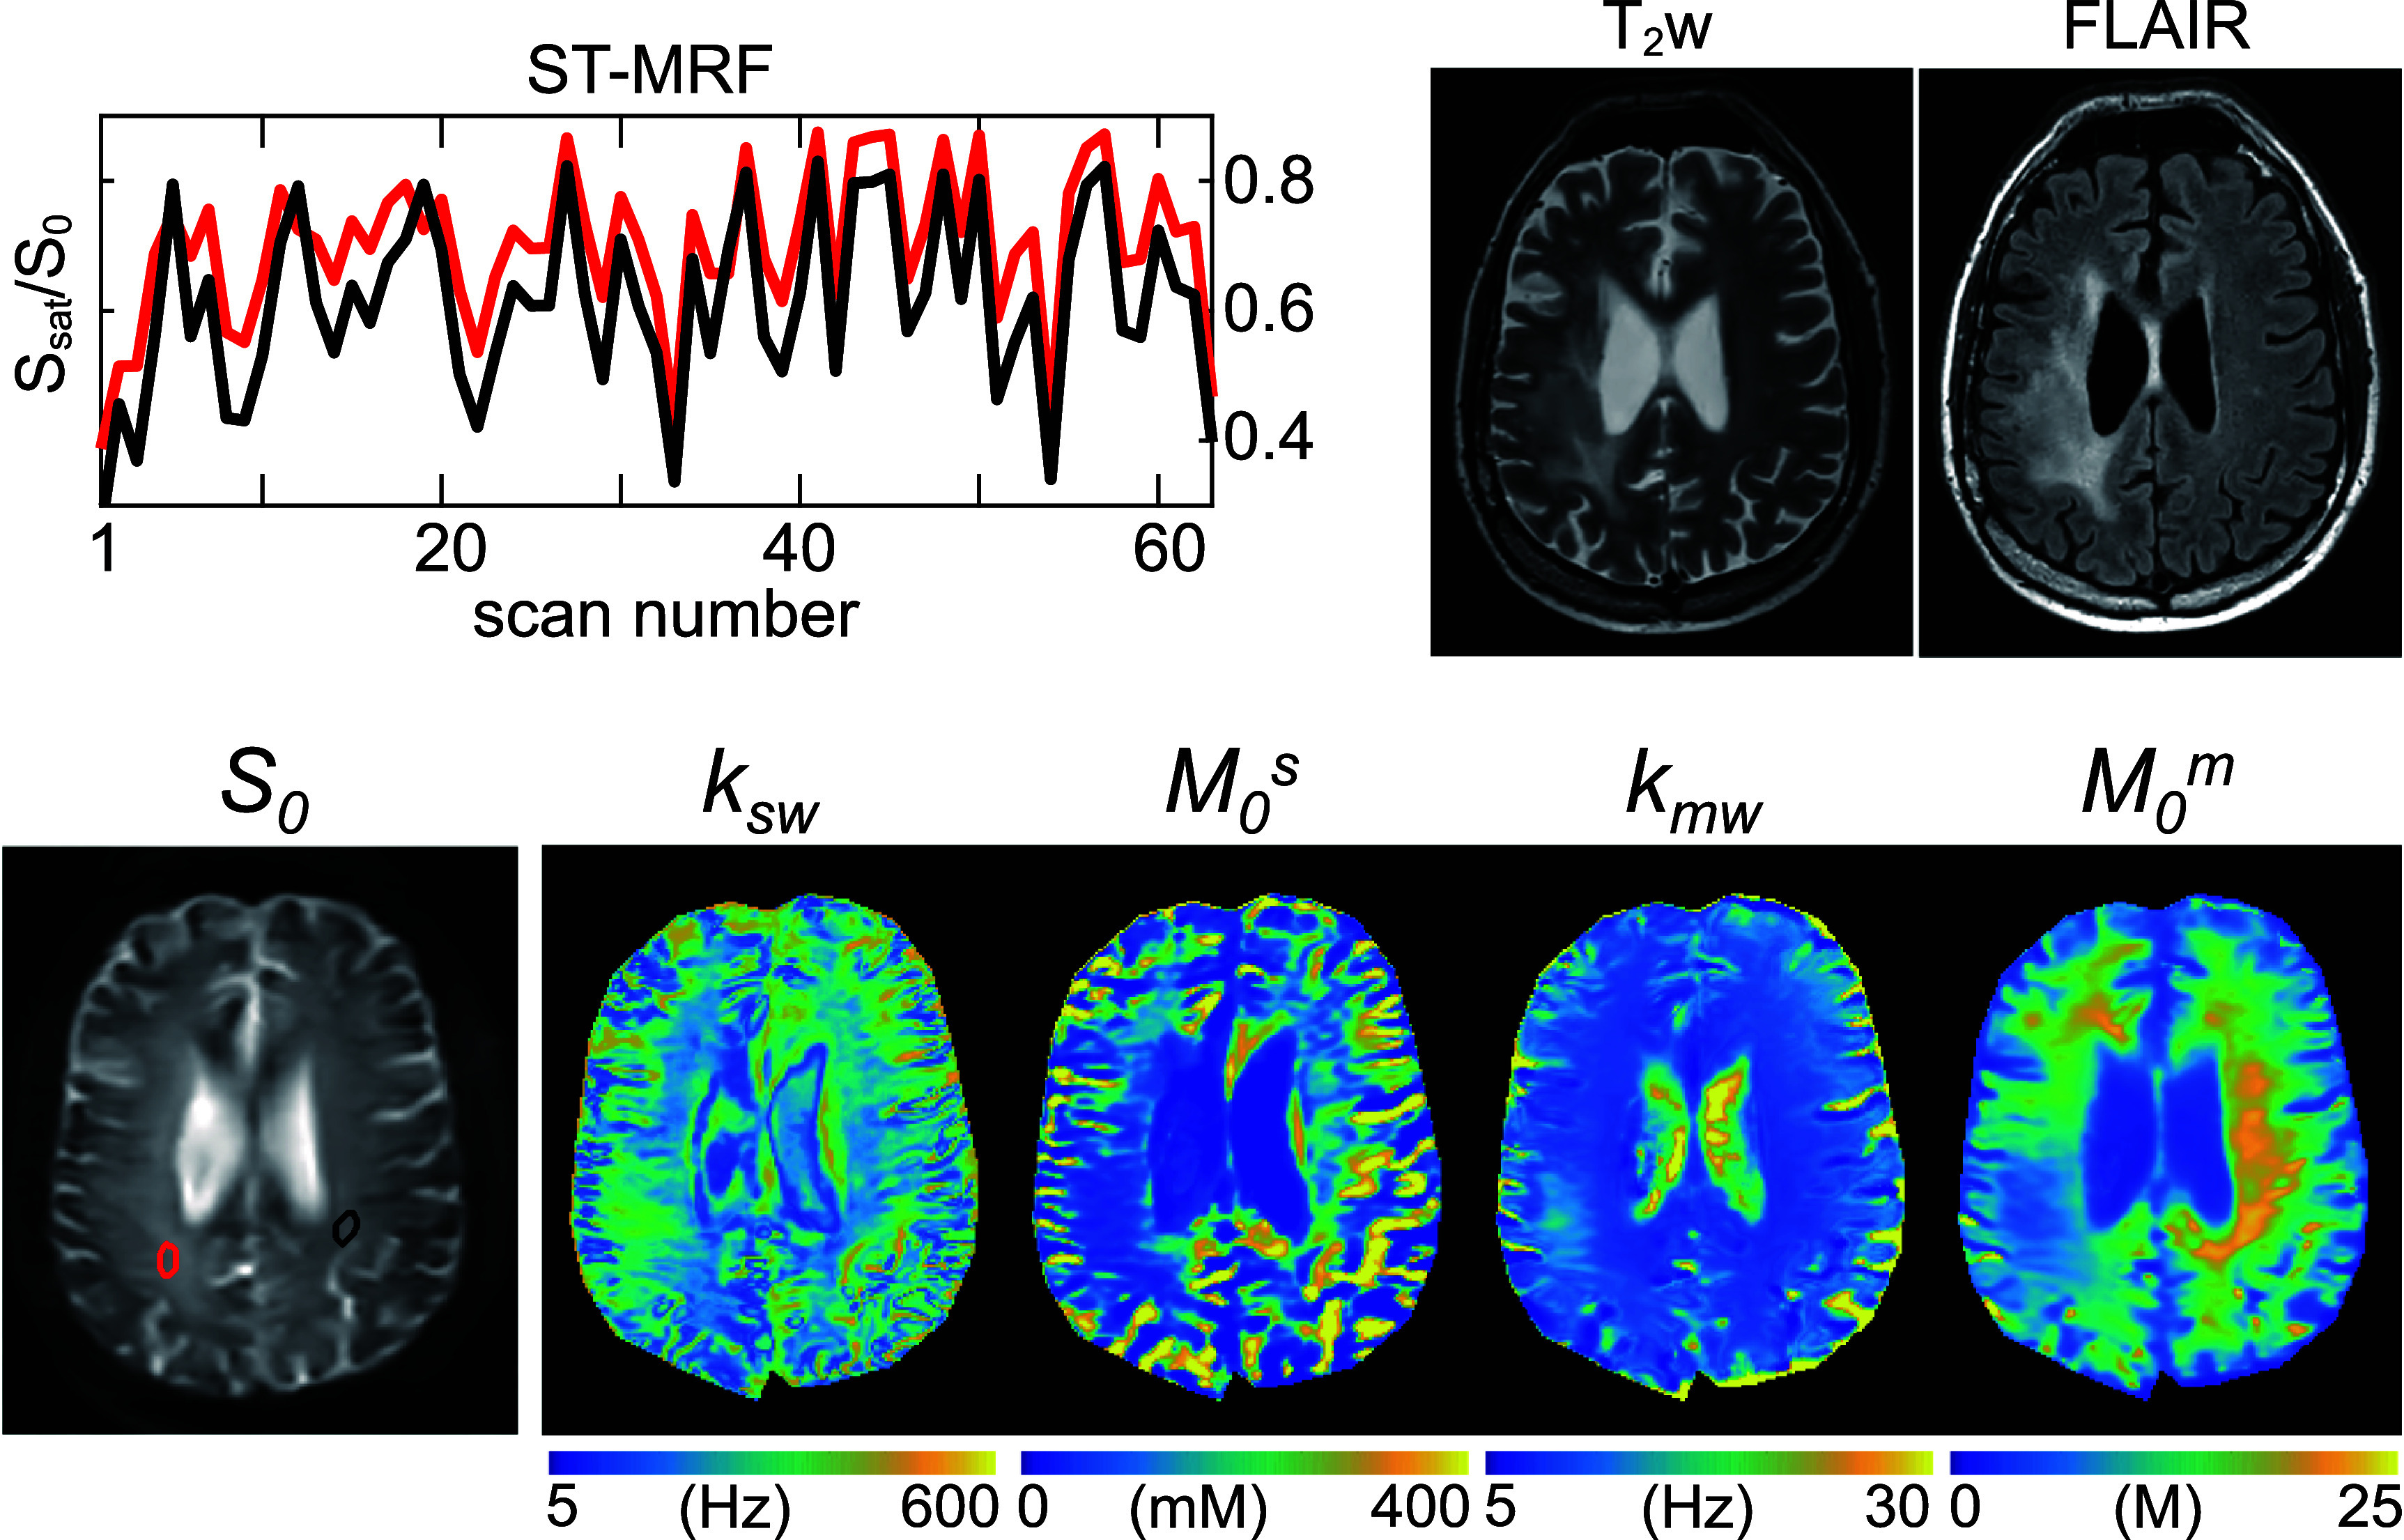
Representative ST-MRF signal evolutions across 63 dynamic scans are shown for the tumor region (red) and the contralateral region (black) in a brain tumor patient with oligodendroglioma. Each ROI is carefully drawn on the corresponding normalization scan (*S*_0_). The estimated quantitative tissue-parameter maps are displayed alongside corresponding *T*_2_-weighted (*T*_2_w) and fluid-attenuated inversion-recovery (FLAIR) images.
